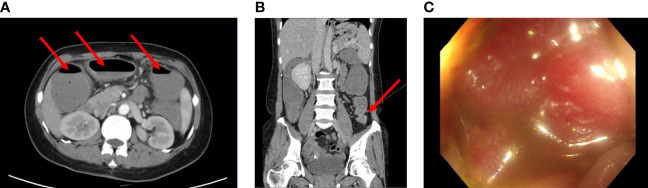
(A, B) Computed tomography images of the patient before surgery (2018-11). Enhanced CT scans of the abdomen revealed the dilatation and gas-fluid level of the colon. (C) Electronic colonoscopy prompts a large cauliflower lump in the colon, situated approximately 70cm from the anal verge. The lesion completely occluded the colon lumen.
